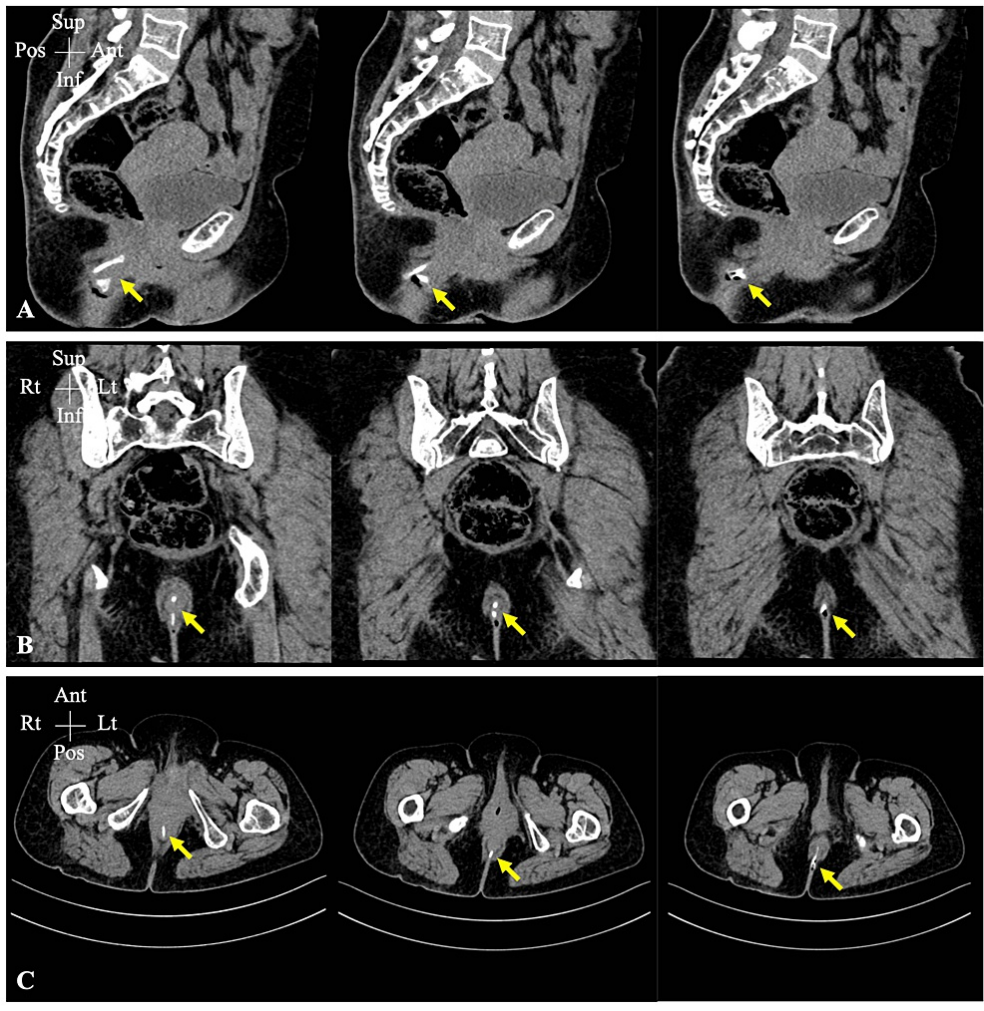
Plain pelvic CT scan Lateral (A), posteroanterior (B), and cross-sectional (C) views of plain pelvic CT scan. The ingested FB was demonstrated (yellow arrow), with the short limb projecting outside the anus, while the long limb was within the anal canal. There were no definite sharp components and no injury to the surrounding structures CT: computed tomography; FB: foreign body; Sup: superior; Inf: inferior; Ant: anterior; Pos: posterior; Rt: right; Lt: left
